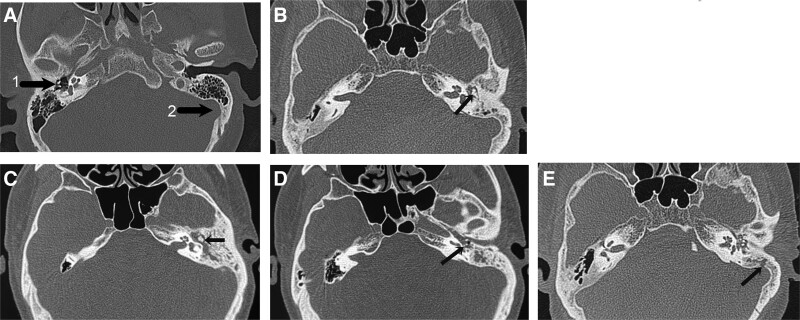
Classification of cholesteatoma according to the degree of bone destruction by CT. (A) CT image of the normal person; intact ossicle chain; intact sigmoid sinus, (B) Those without obvious destruction of auditory ossicles were graded as (+), (C) Those with destruction of incus or malleus were graded as (+ +), ↖pointed to the malleus and incus, (D) Those with destruction of incus and stapedius were graded as (+ + +), ↖ pointed to the stapedius, and (E) Those with destruction of incus, malleus, mastoid tegument and/or sigmoid sinus bone plate were graded as (+ + + +), ↖ pointed to the sigmoid sinus bone plate. (WW 3000–4000HU, WL 350–450HU).
